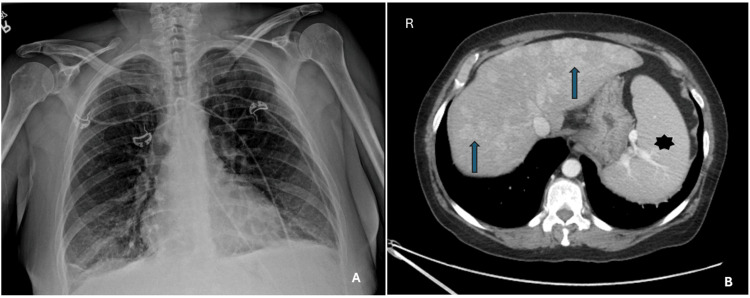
Imaging findings in a 56-year-old female with chest pain, anemia, and thrombocytopenia. (A) Chest x-ray, frontal view, showing hyperexpanded lungs, mild bibasilar linear opacities with no distinct pleural effusion and no pneumothorax. (B) CT scan of the abdomen showing multiple liver nodules (arrows), hepatomegaly, and splenomegaly (star)
